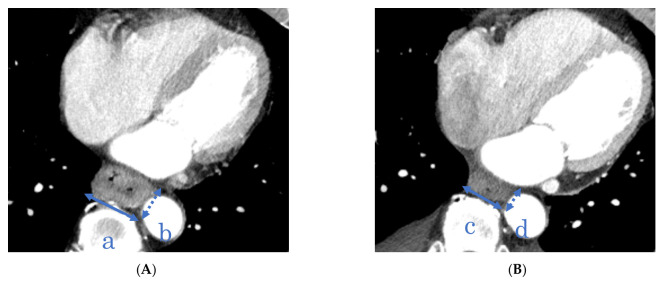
Assessment of long- and short-axis diameters on contrast-enhanced computed tomography before and after chemotherapy. (A) Computed tomography image before chemotherapy. The long-axis diameter is indicated by a solid arrow (a), and the short-axis diameter is indicated by a dotted arrow (b). (B) Computed tomography image of the same slice after chemotherapy. The long-axis diameter is indicated by a solid arrow (c), and the short-axis diameter is indicated by a dotted arrow (d).
